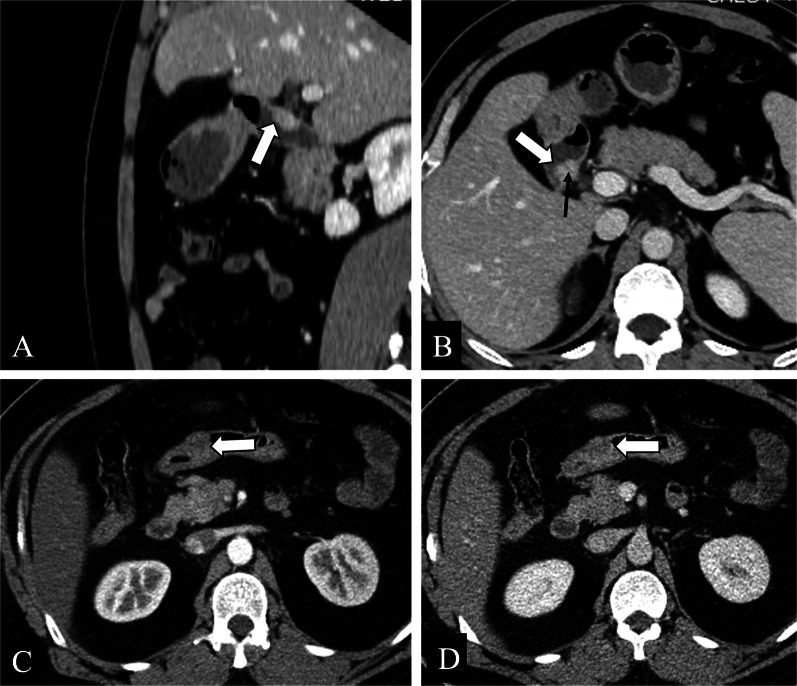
a, b Coronal and axial enhanced CT images of a heterotopic pancreas in the duodenum of a 53-year-old male showed a 1.3 cm × 0.9 cm, endoluminal lesion (white arrows) with a lobulated margin, presenting round contour on axial image while flat pattern on coronal CT image. Small duct-like structure (black arrow) was seen within the lesion, which measured with a long diameter to short diameter > 1.4 on coronal CT image. c, d Axial enhanced CT images of a heterotopic pancreas in the gastric antrum of a 58-year-old male showed a 1.2 cm × 0.8 cm, oval, ill-defined, and endoluminal lesion (white arrows), with a long diameter to short diameter > 1.4
